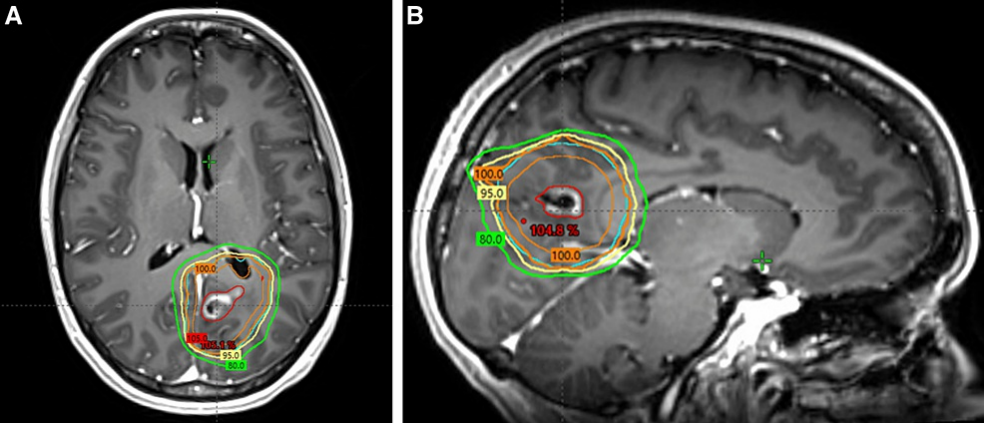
Radiation dose distribution. Radiation dose distribution demonstrating the gross tumor volume (red), clinical target volume (orange), and planning target volume (cyan). The 100% (orange), 95% (yellow), and 80% (green) isodose lines are shown on the pre-treatment axial (A) and sagittal (B) T1 MRI with contrast.
